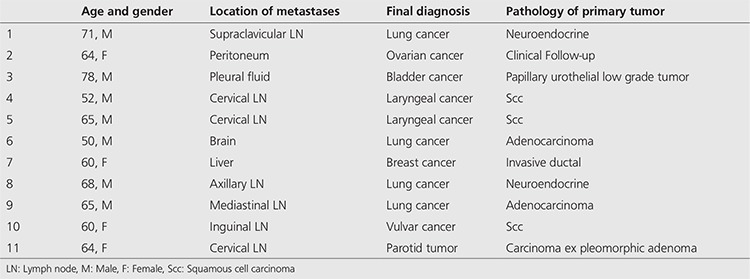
False-negative results of ^18^F-fluorodeoxyglucose positron emission tomography/computed tomography in patients with carcinoma of unknown primary
